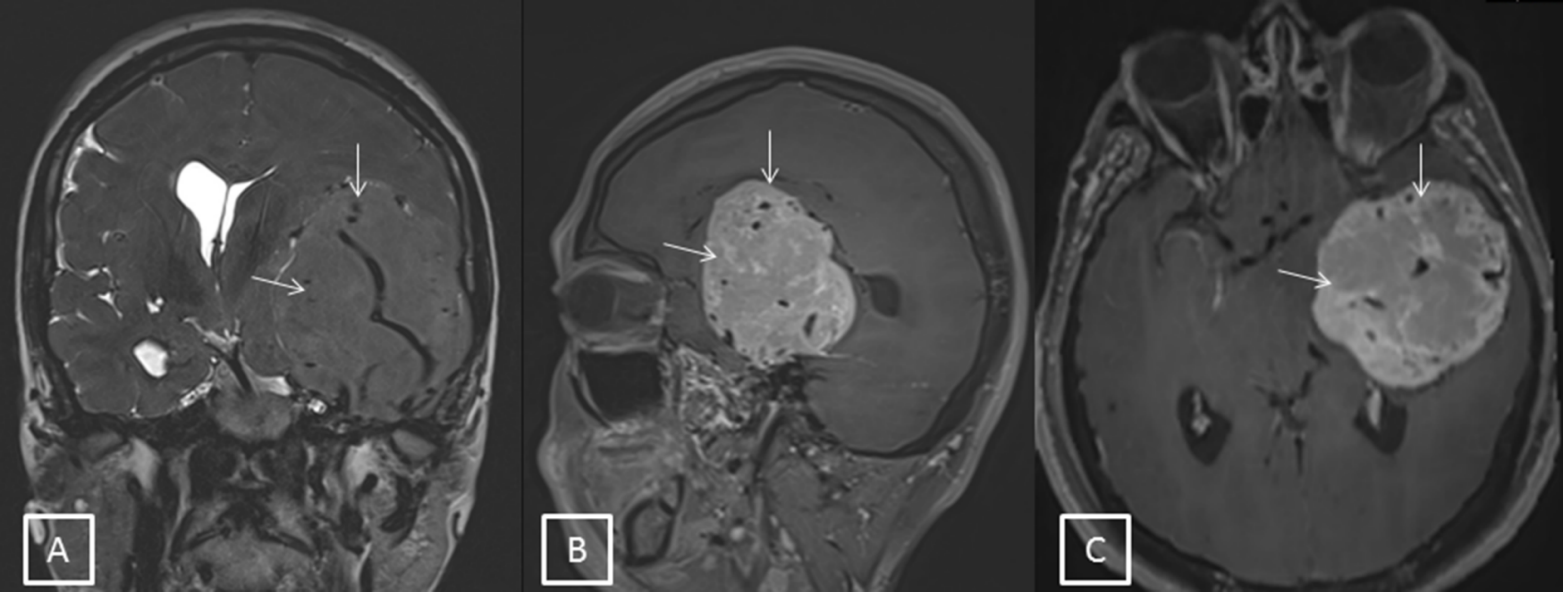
A) Coronal T2-weighted MRI brain showing an iso-hyperintense large mass lesion in the tempo-parietal lobe (white arrows). B,C): Post contrast sagittal and axial cuts showing an intense heterogeneous enhancement of the lesion (white arrows).
